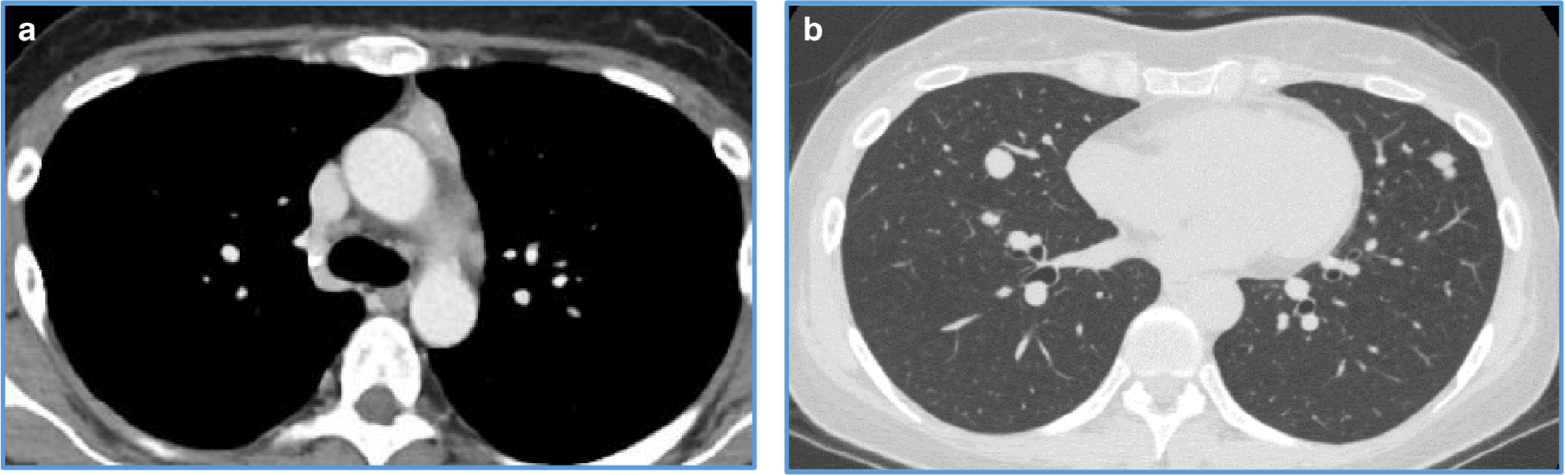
Computed tomography (CT) showed an anterior mediastinal mass (a), mediastinal lymph node and multiple pulmonary nodules (b).
